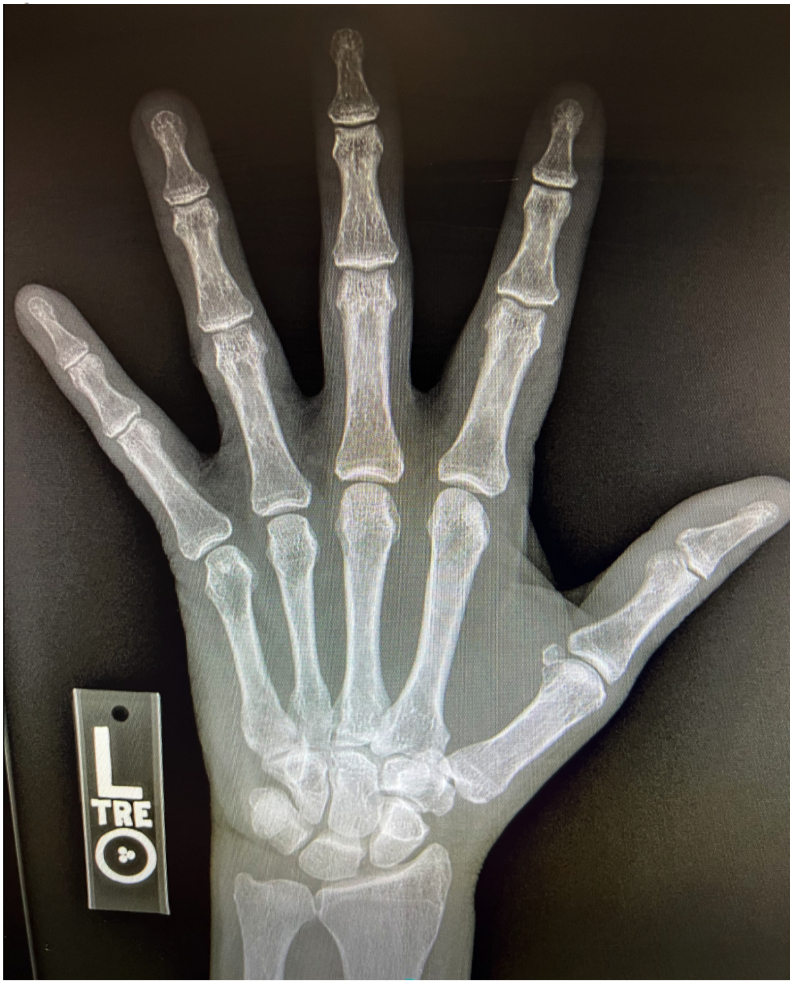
Radiographic image of hand.
